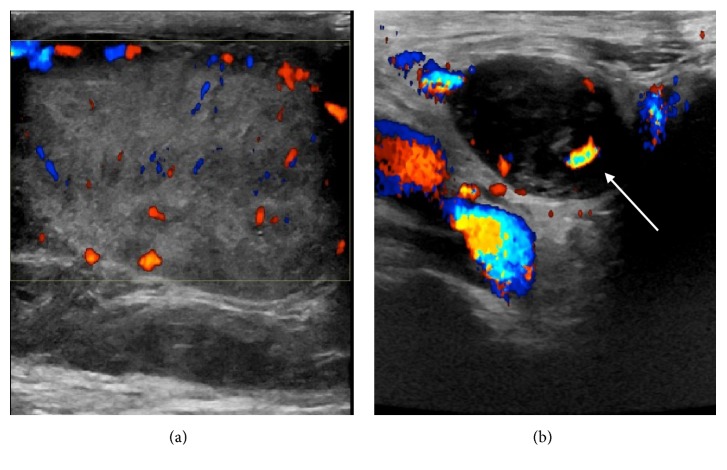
(a) Ultrasound of the scrotum demonstrates an enlarged diffusely heterogeneous right testicle and epididymis with areas of increased vascularity. (b) Ultrasound of the right spermatic cord at the level of the right inguinal canal. Image demonstrates a tubular predominantly hypoechoic mass with internal vascularity (white arrow).
